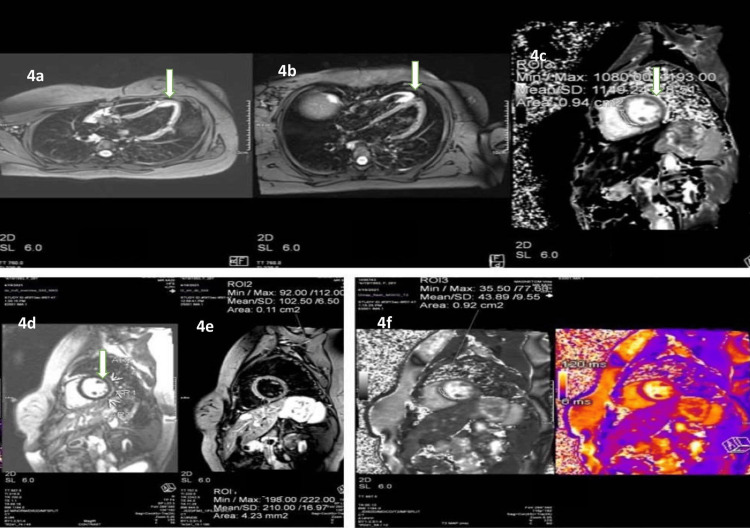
Cardiac magnetic resonance imaging showed hyperintensity signal changes in pericardium and myocardium
